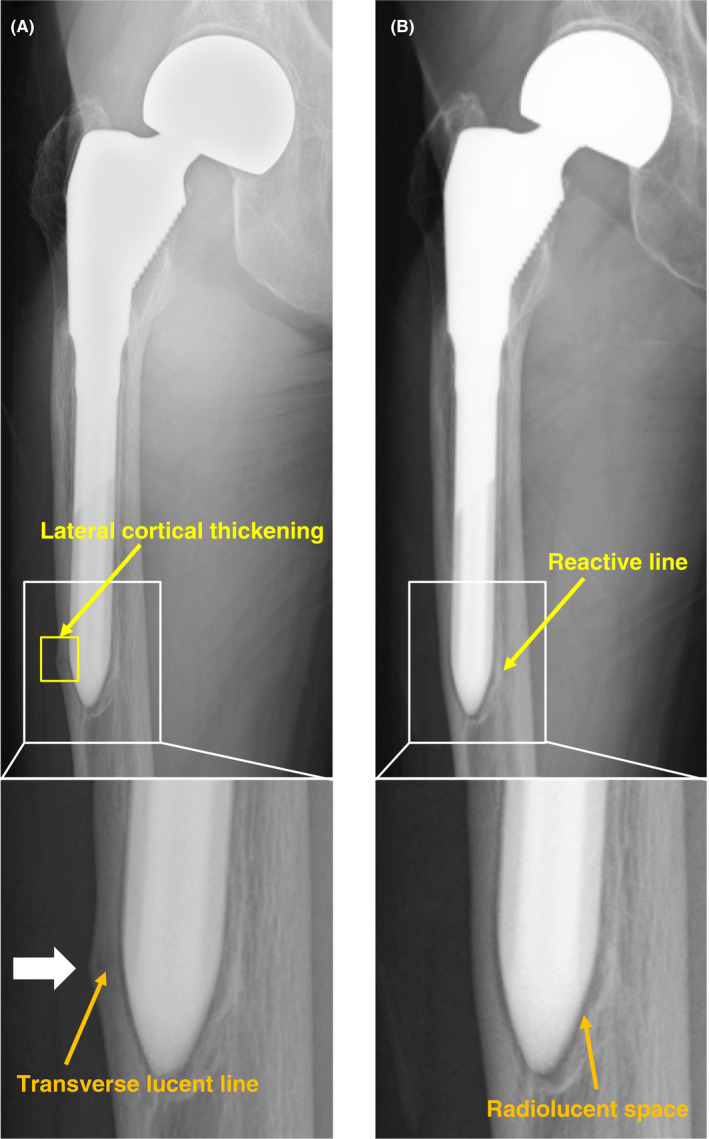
Varus femoral component with beaking and an obscure transverse lucent line a little above the stem tip. A, Frontal femur radiography obtained when the patient first visited to our hospital complaining of right severe thigh pain showed a lucent area around the stem with subtle thickening and beaking of the lateral cortex near the distal stem tip (white arrow). A higher magnification image of the framed area is shown in the lower panel. Yellow and orange arrows indicate lateral cortical thickening and an obscure transverse lucent line, respectively. B, Radiograph obtained 3 y earlier showed no periosteal thickening of the lateral cortex a little above the stem tip. A higher magnification image of the framed area is shown in the lower panel. Yellow and orange arrows indicate a reactive line and a radiolucent space, respectively
